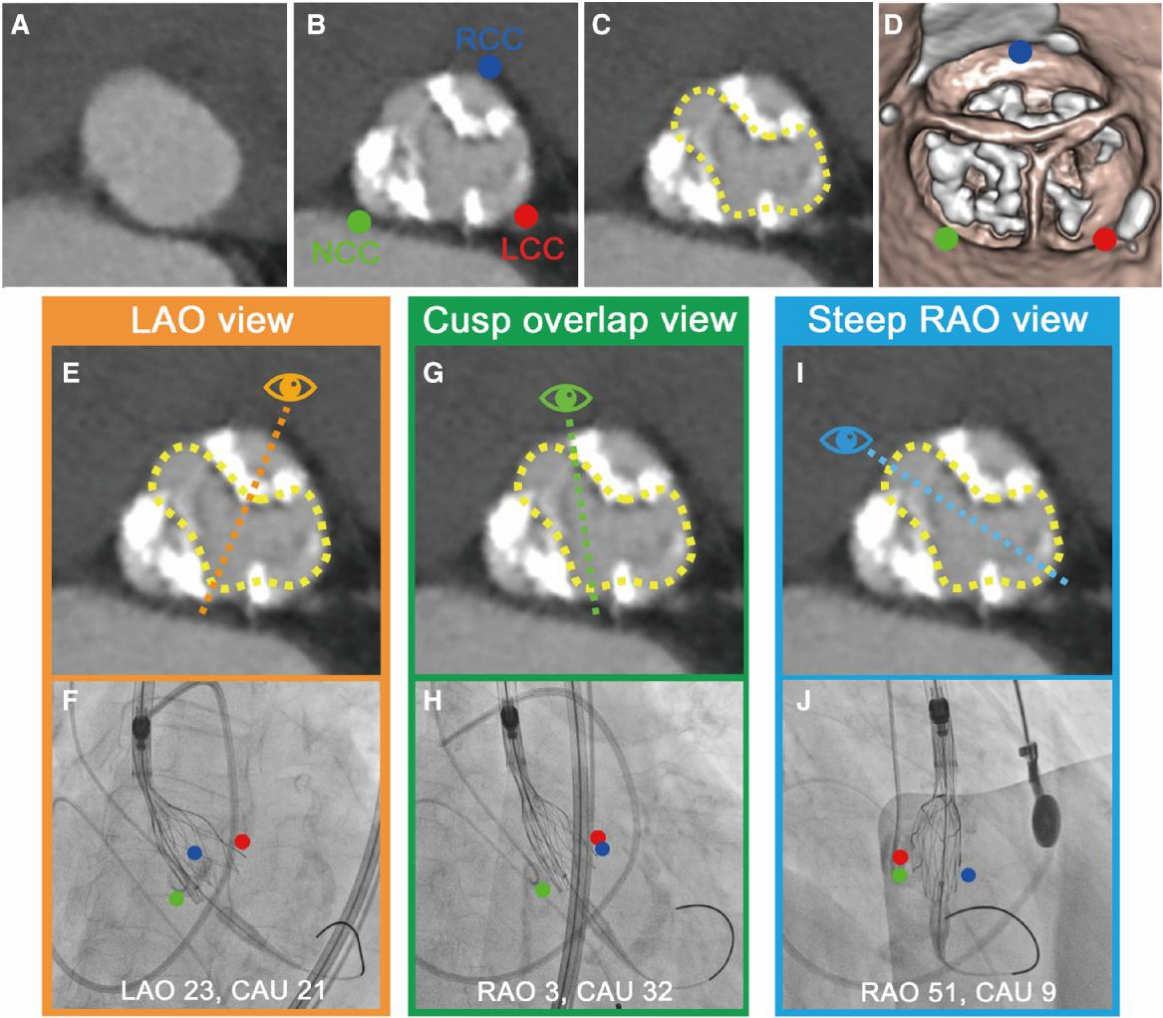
Comparison of the left anterior oblique, cusp-overlap, and steep right anterior oblique views. (*A–D*) Pre-procedural computed tomography imaging showing heavy calcification, especially in the right- and non-coronary cusps. (*A*) Baseline annulus. (*B*) Uneven distribution of heavy calcification in the three cusps. (*C*) Theoretically predicted shape of a transcatheter heart valve that is partially deployed and under-expanded (yellow-dotted line). (*D*) Volume-rendering image. (*E* and *F*) Left anterior oblique view observed by an operator in the direction of the dotted orange line. (G and *H*) An operator views the cusp-overlap view towards the dotted green line. (*I*) Steep right anterior oblique view observed by an operator in the direction of the dotted blue line. (*J*) Only the steep right anterior oblique view elucidates the constrained transcatheter heart valve. The blue circle represents the right-coronary cusp; the red circle represents the left-coronary cusp; and the green circle indicates the non-coronary cusp.
